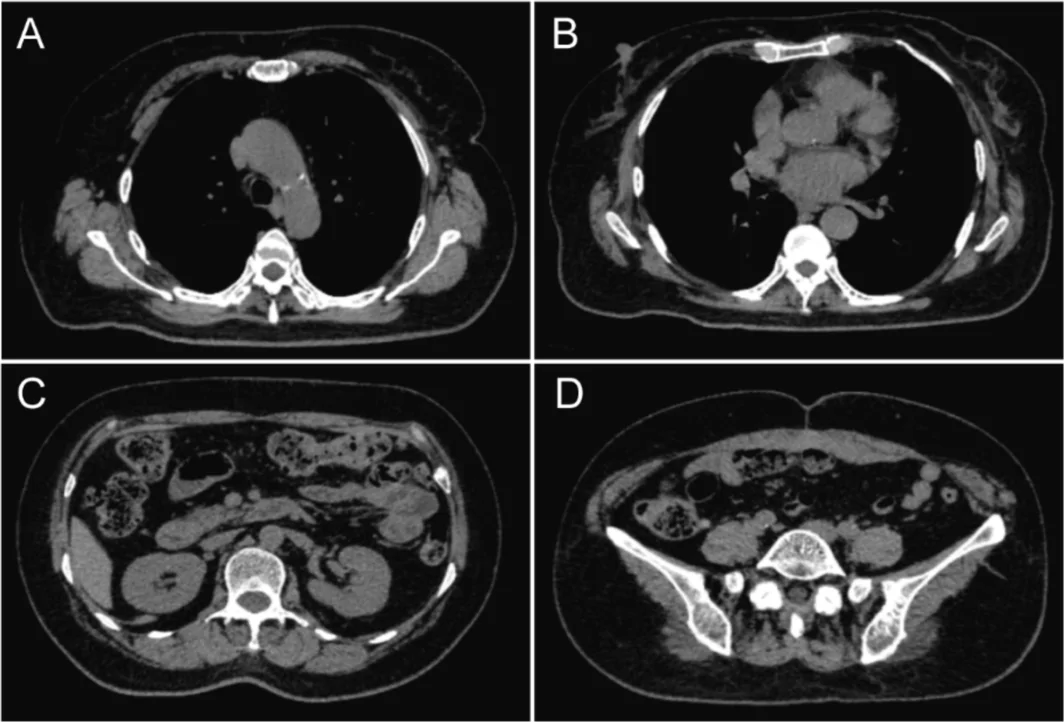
Follow‐up CT images showing no obvious enlarged lymph nodes or radiologic evidence of disease progression. (A) Chest CT showing no obvious enlarged parasternal lymph nodes. (B) Chest CT showing no obvious recurrent breast lesion or axillary lymphadenopathy. (C) Abdominal CT showing no obvious enlarged abdominal or retroperitoneal lymph nodes. (D) Pelvic CT showing no obvious enlarged pelvic lymph nodes.
